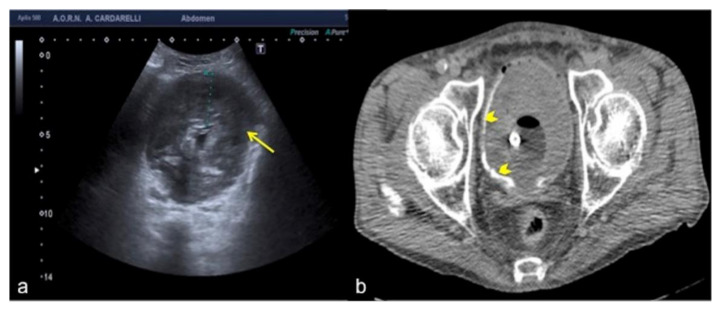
Transverse US scan of the bladder (a) shows a diffuse thickening of the wall (arrow) in a 78-year-old man investigated for hematuria. On CT scan (b) it appeared to be a large blood clot occupying the entire lumen of the bladder (arrowheads).
